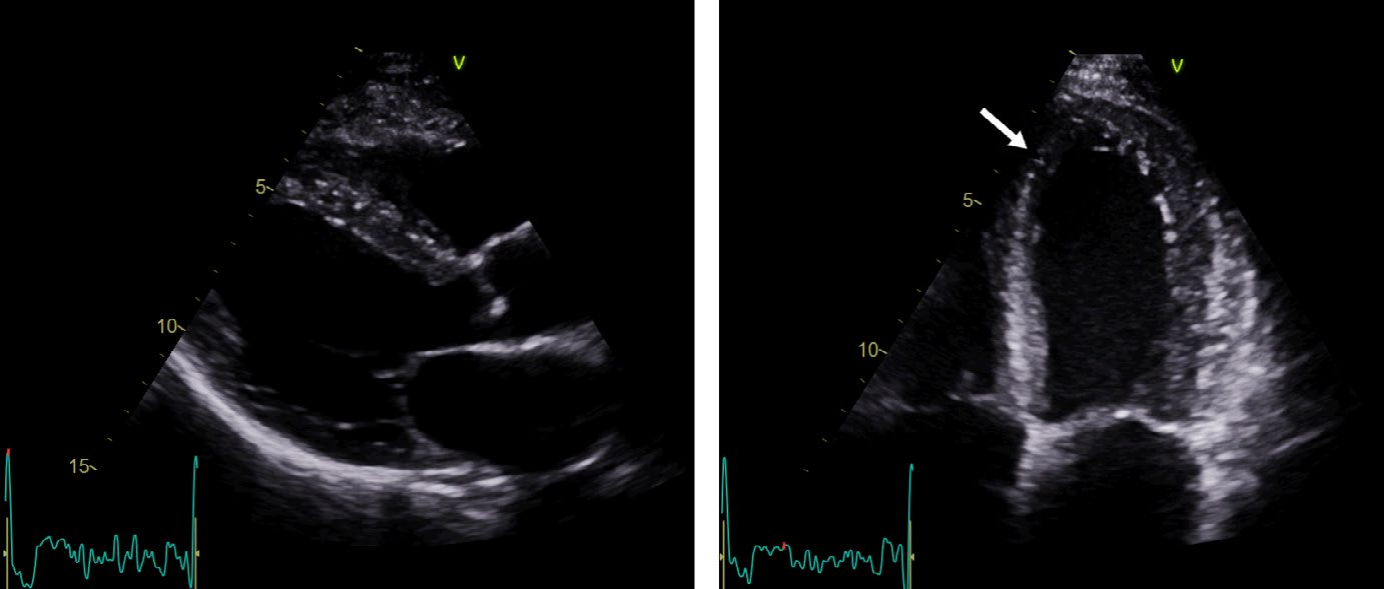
Left hand panel shows a end‐diastolic parasternal long axis echo image. No left ventricular hypertrophy or valve disease was found. Right hand panel shows a end‐systolic apical 4 chamber view with anteroseptal hypokinesia (white arrow)
